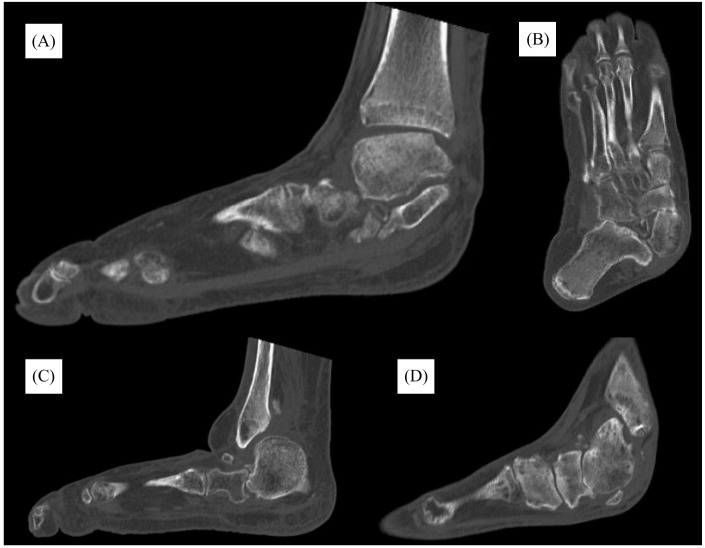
Computed tomography with (A,C,D) multiple sagittal cuts, and (B) a coronal cut after follow-up in orthopedic clinic demonstrating findings consistent with advanced stage Charcot joint with marked pes planus, mid-foot collapse, and hindfoot valgus.
